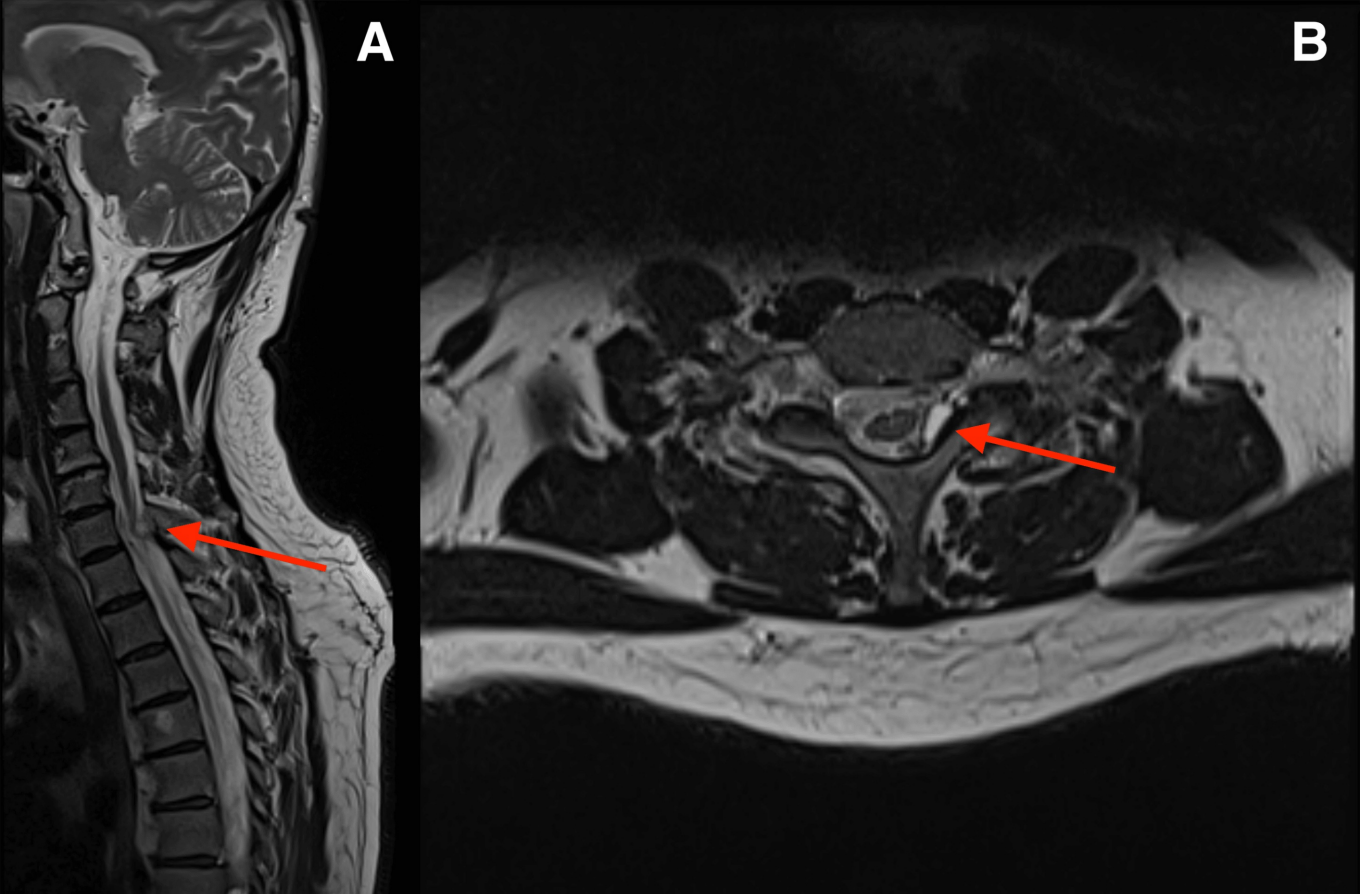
Cervical spine MRI A: Sagittal MRI showing a cervical synovial cyst at the C7-T1 level (red arrow). B: Axial MRI demonstrating an intraspinal, extramedullary cyst located left parasagittally, causing spinal cord compression and associated myelopathy (red arrow). MRI: magnetic resonance imaging
